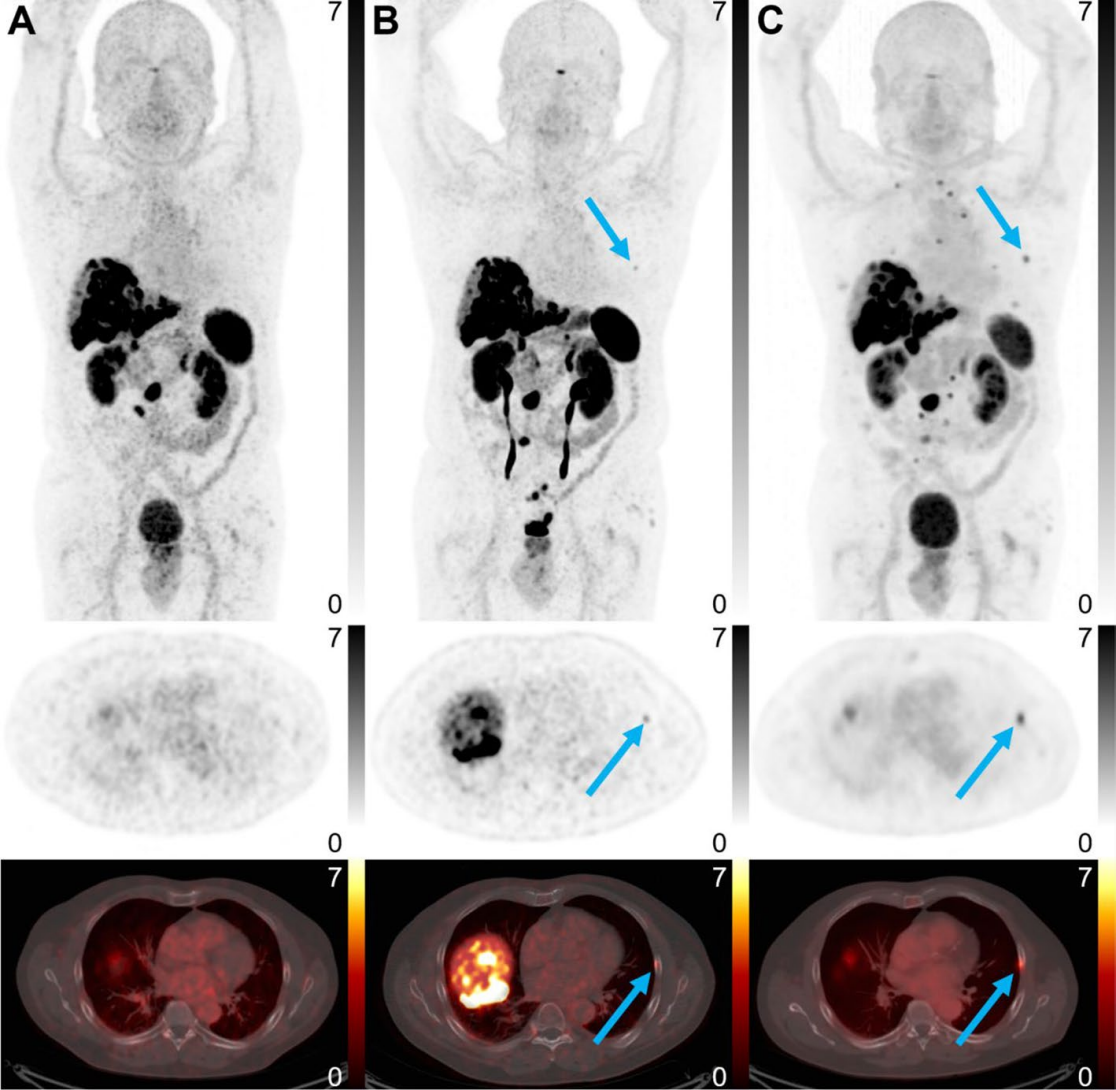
A ^68^Ga-DOTANOC and B ^18^ F-AlF-OC images (from top to bottom: maximum-intensity projection PET, transversal PET and fused PET/CT images, respectively) of a seventy-year old male patient with an intestinal neuroendocrine tumor with multiple liver metastases and one involved lymph node. ^18^ F-AlF-OC detected an additional bone lesion (blue arrow) that was confirmed by C follow-up ^68^Ga-DOTANOC imaging 6 months later. Lookup tables apply to PET images (SUV). (From Pauwels et al. 2022)
